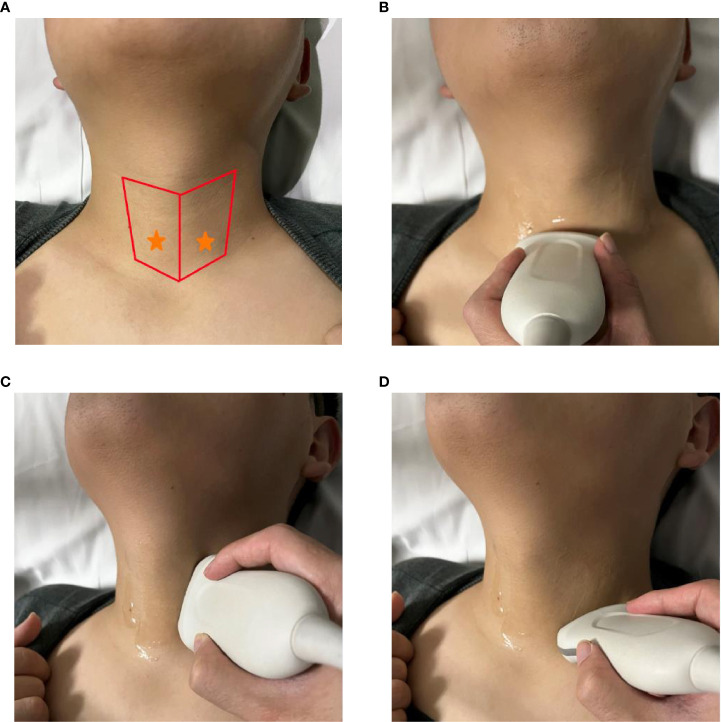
Identification of normal Inferior parathyroid glands using ultrasonography. (A) red area: main scanning range, yellow star: expected location of IPGs; (B–D) operation method of scanning IPGs.
